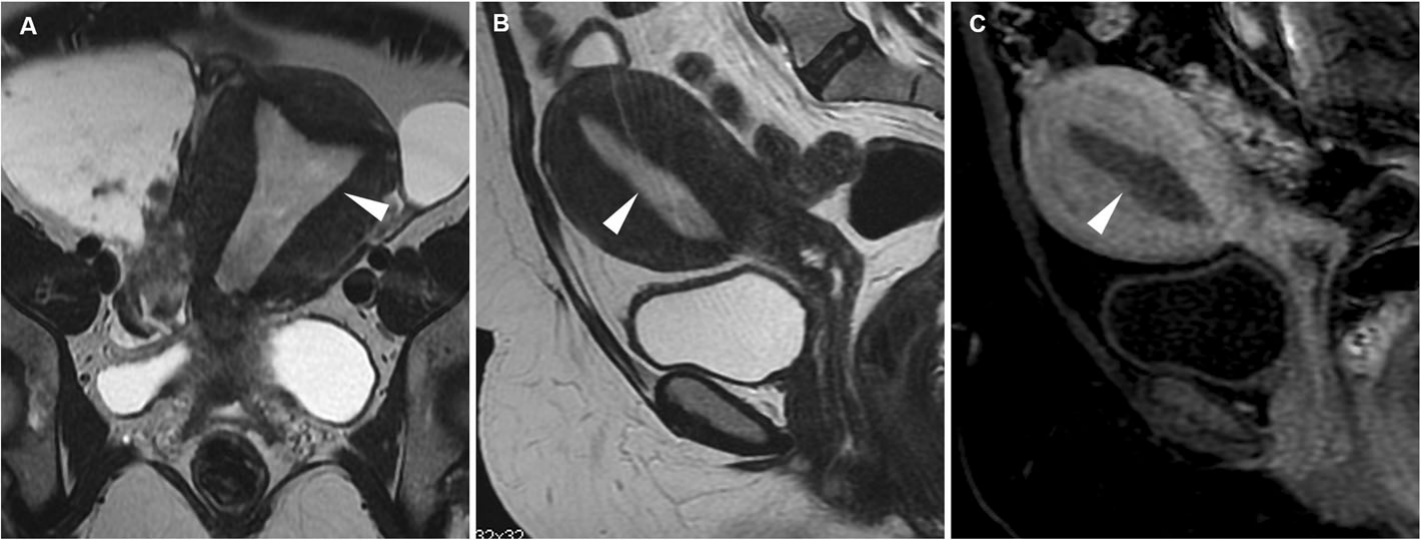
Endometrial hyperplasia in a 43-year-old woman with acute abnormal uterine bleeding. Oblique-coronal (a), sagittal (b) T2-weighted images and gadolinium-enhanced sagittal (c) fat-suppressed T1-weighted image show marked endometrial thickening (arrowheads) without macroscopic signs of myometrial invasion. Hysteroscopy with endometrial biopsy demonstrated simple endometrial hyperplasia without atypia
